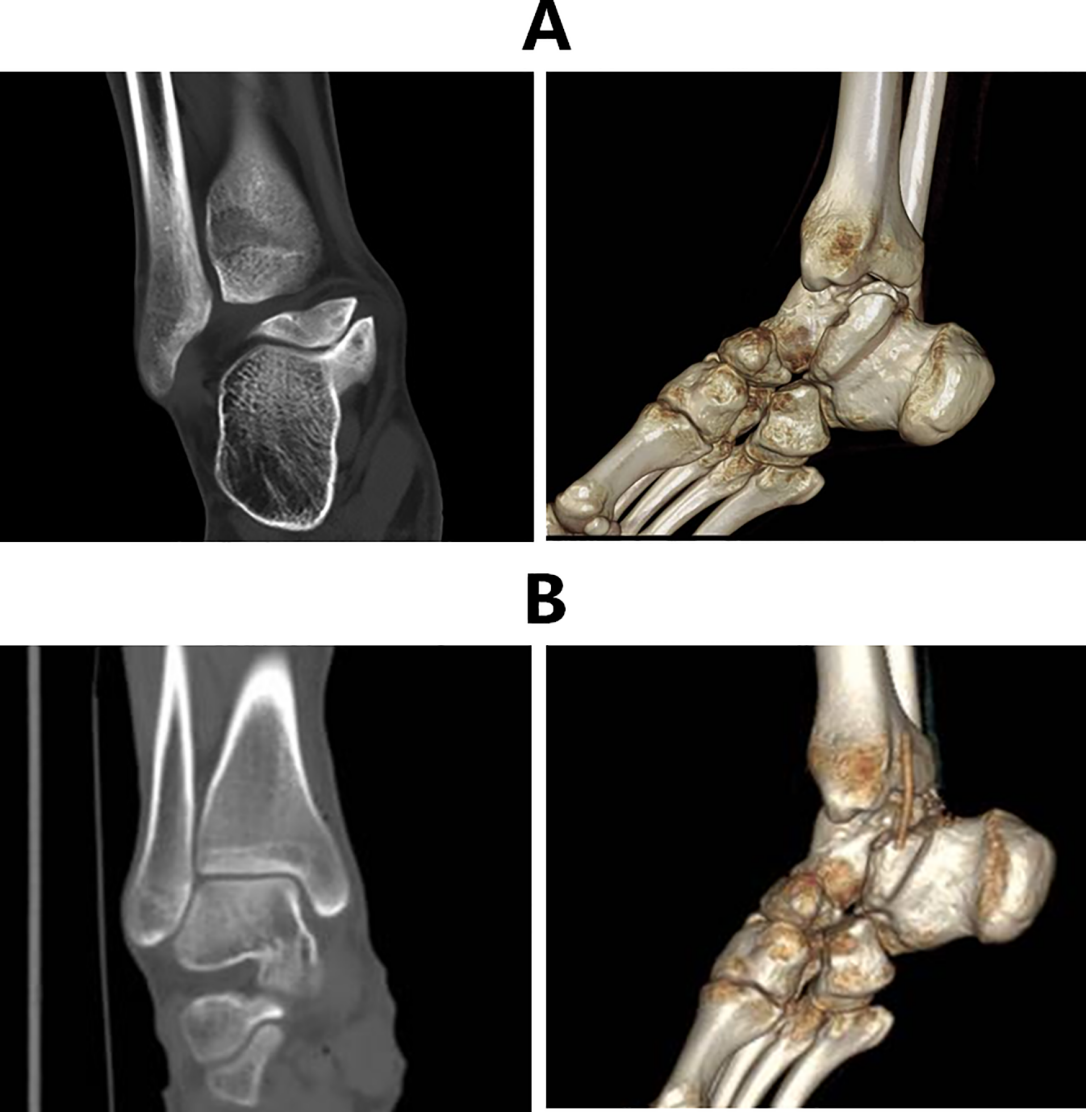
CT of TCC pre- (A) and post-operation (B).
